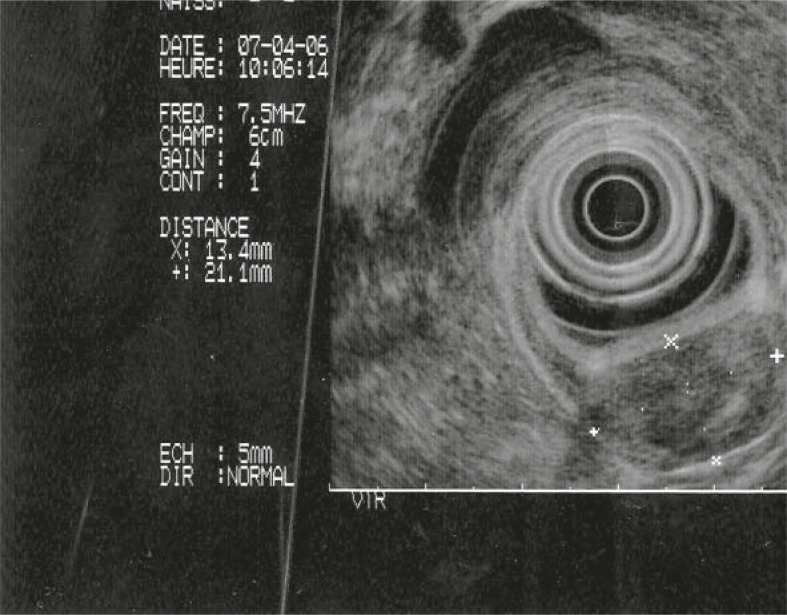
Endoscopic ultrasonography revealed a submucosal polypoid mass located at the anterior surface of duodenal bulb.
